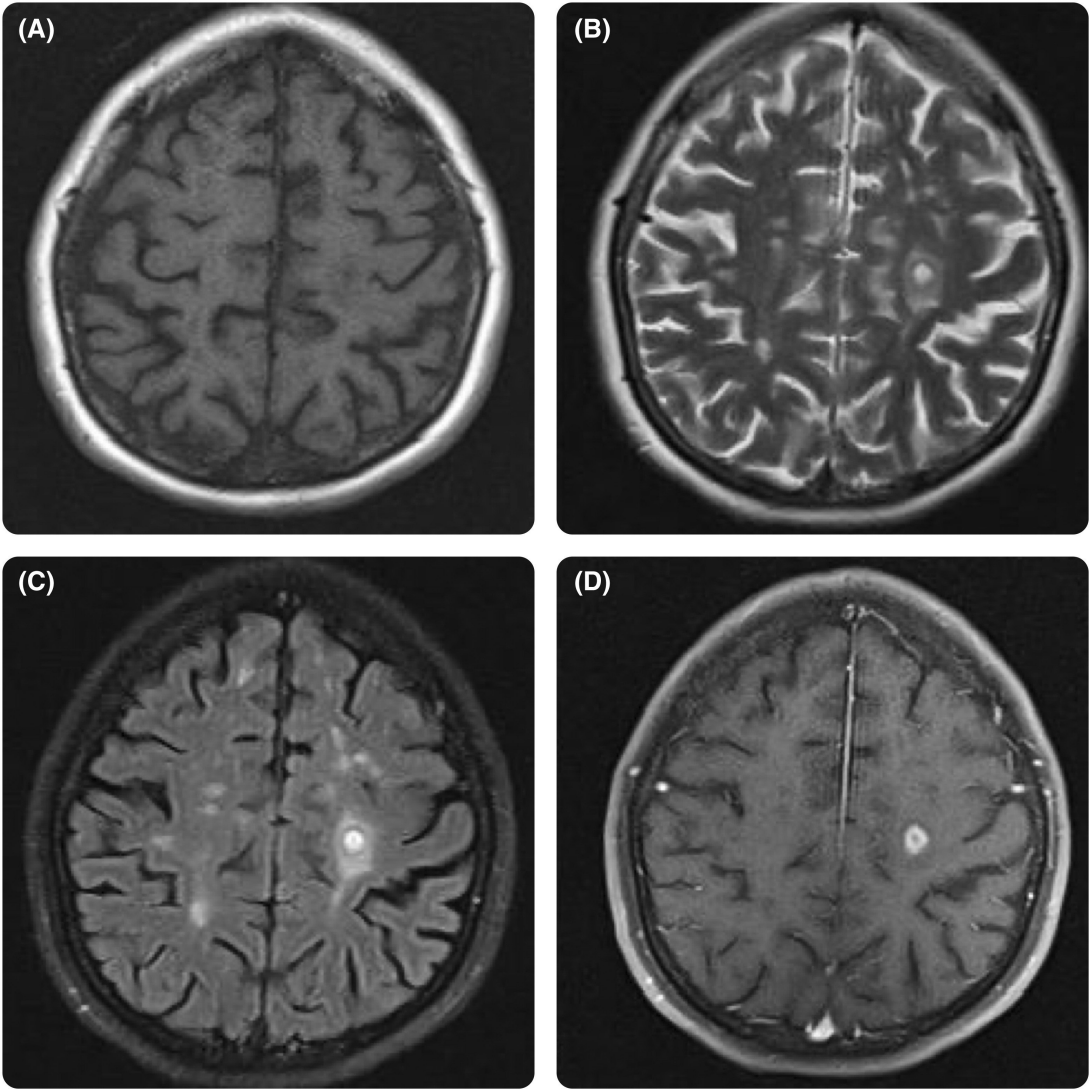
(A) Brain MRI T1‐weighted image showed multiple hypointense lesions. (B, C) T2‐ and flair revealed multiple hyperintense lesions. One of them located in left centrum semiovale had multiple hypo‐ and hyperintense layers, (D) with closed ring enhancement. MRI, magnetic resonance imaging.
